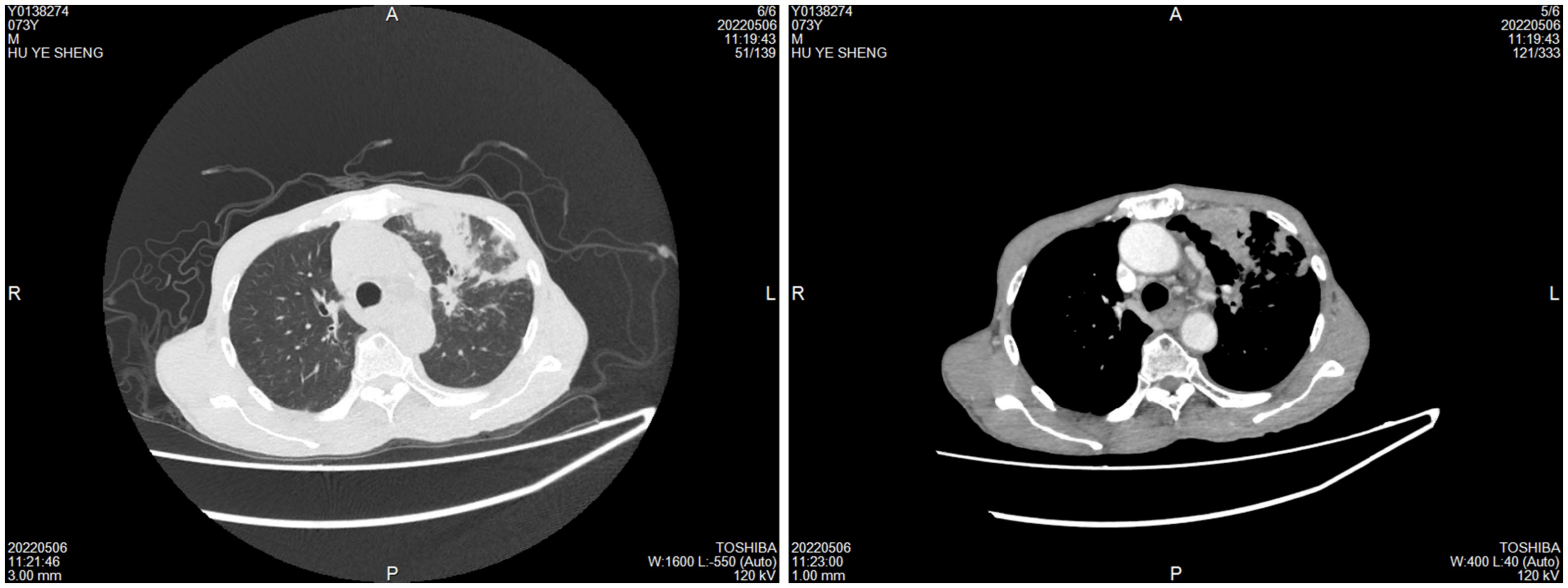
Imaging examination of pulmonary tuberculosis. CT scans revealed lobular and nodular high-density shadows, with cavity shadows observed in some lesions.
